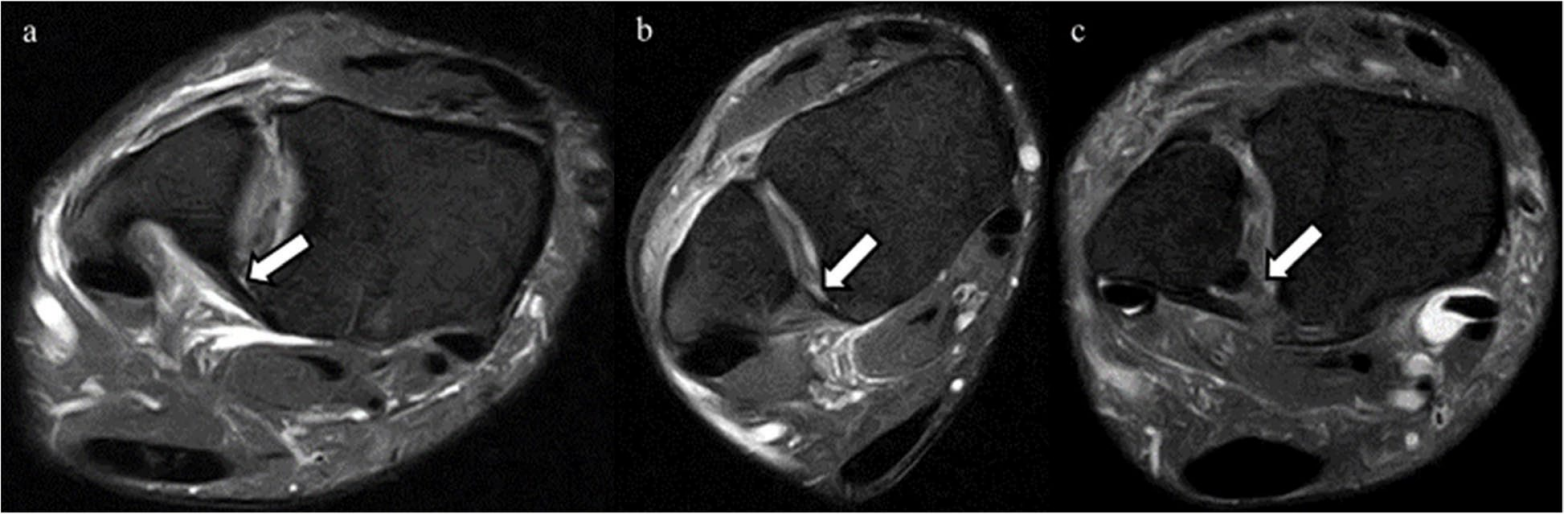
Transverse ligament (TL) in oblique axial proton-density fat saturation MRI. The arrows in the figures indicate TL. a The intact TL. b Partial tear of the TL, with hyperintensity of the ligament. c Complete tear of the TL, showing complete discontinuity
